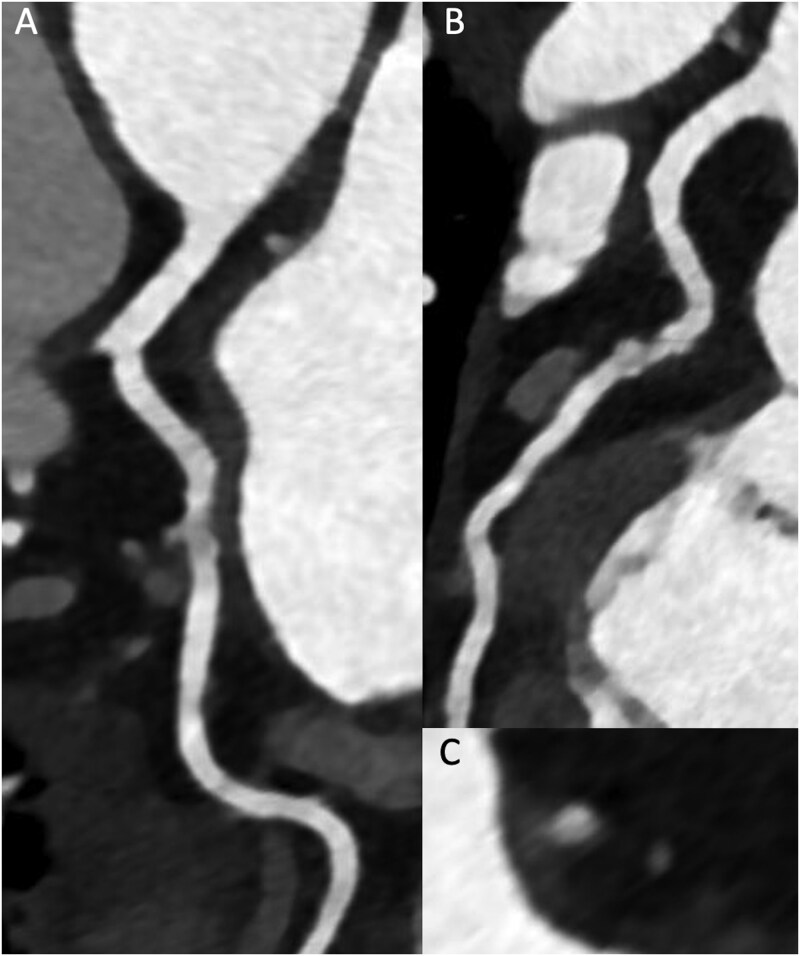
The limitations of the FLASH protocol. The panels (A-C) show reduced luminal contrast due to possible mixed plaque at the proximal LCx. A horizontal motion artifact across in the segment of interest prevented detailed assessment. A moderate luminal stenosis seen on CT was actually severe on the invasive angiogram.
